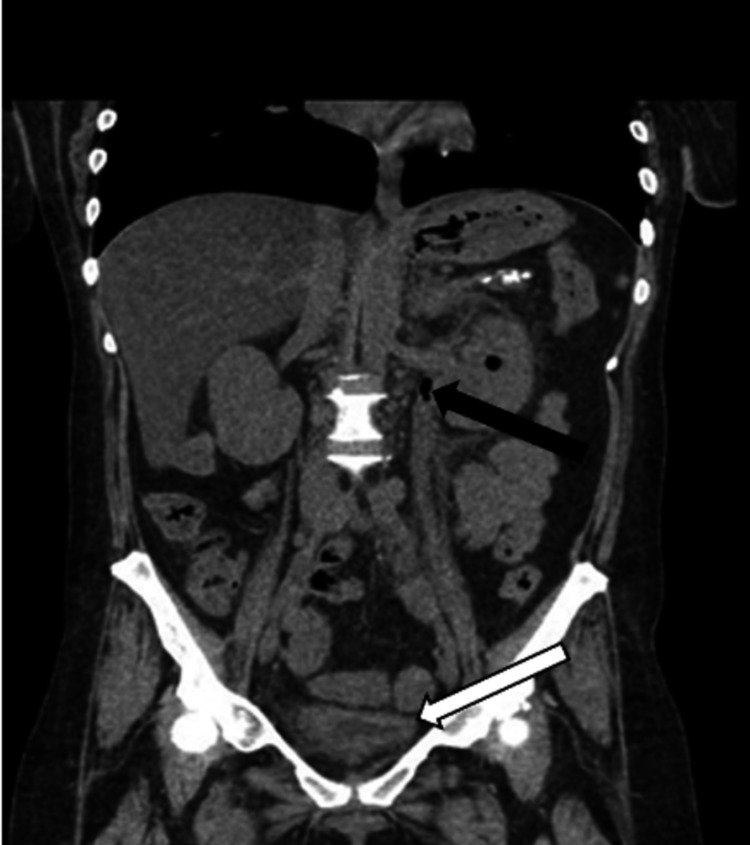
CT of the abdomen and pelvis without contrast Abdominal CT demonstrating gas in the renal collecting system, with the black arrow demonstrating gas in the ureter with hydroureter and the white arrow showing thickened bladder wall with perivesicular edema.
